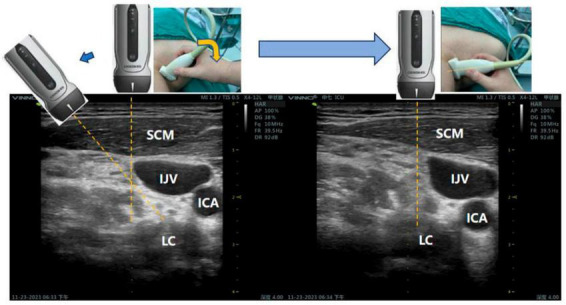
Ultrasonic probe adjustment method: The dashed yellow line represents the median line of the ultrasound probe and served as a guide for the puncture. IJV, internal jugular vein; ICA, internal carotid artery; LC, longus colli muscle; SCM, sternocleidomastoid muscle.
